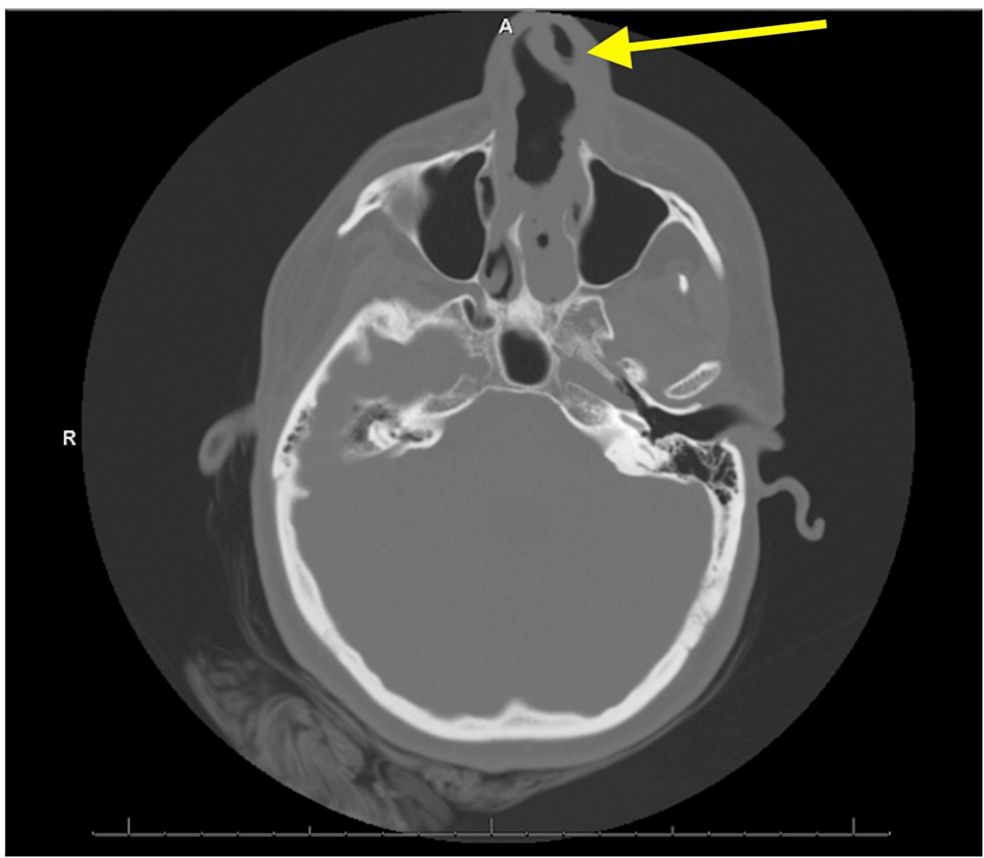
Brain CT without contrast The radiologist noted no acute intracranial abnormality but a perforated nasal septum with soft tissue thickening of the nasal region; please refer to the dedicated CT of the facial bones.
